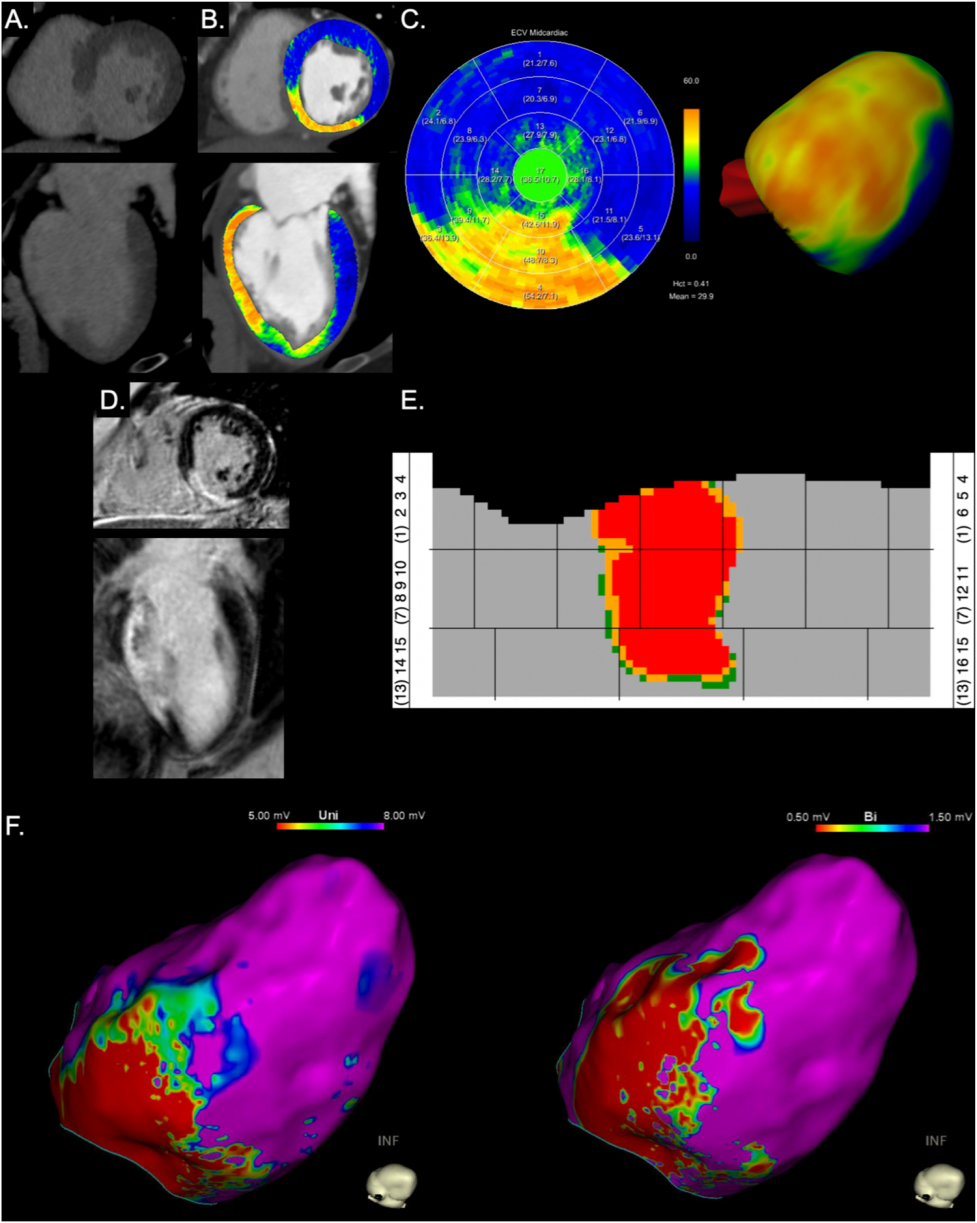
Representative images of the left ventricle of a 62-year-old female patient with ischemic cardiomyopathy. Virtual monoenergetic images (at 65 keV) of cardiac late enhancement photon-counting detector CT show iodine enhancement of the inferior wall of the left ventricle (A). Corresponding high extracellular volume (ECV) is demonstrated in coronary CT angiography with an overlay visualizing ECV (B), as well as in ECV polar maps (C) and atlas maps (E). Prior cardiac MR imaging shows identical extent of late gadolinium enhancement (D) to the ECV elevation observed in late enhancement CT. Endocardial electroanatomic mapping (EAM) (F) shows myocardial low-voltage areas of the inferior/inferolateral basal and inferio/inferoseptal midventricular segments in unipolar (< 5.0 mV) and bipolar (< 0.5 mV) EAM
